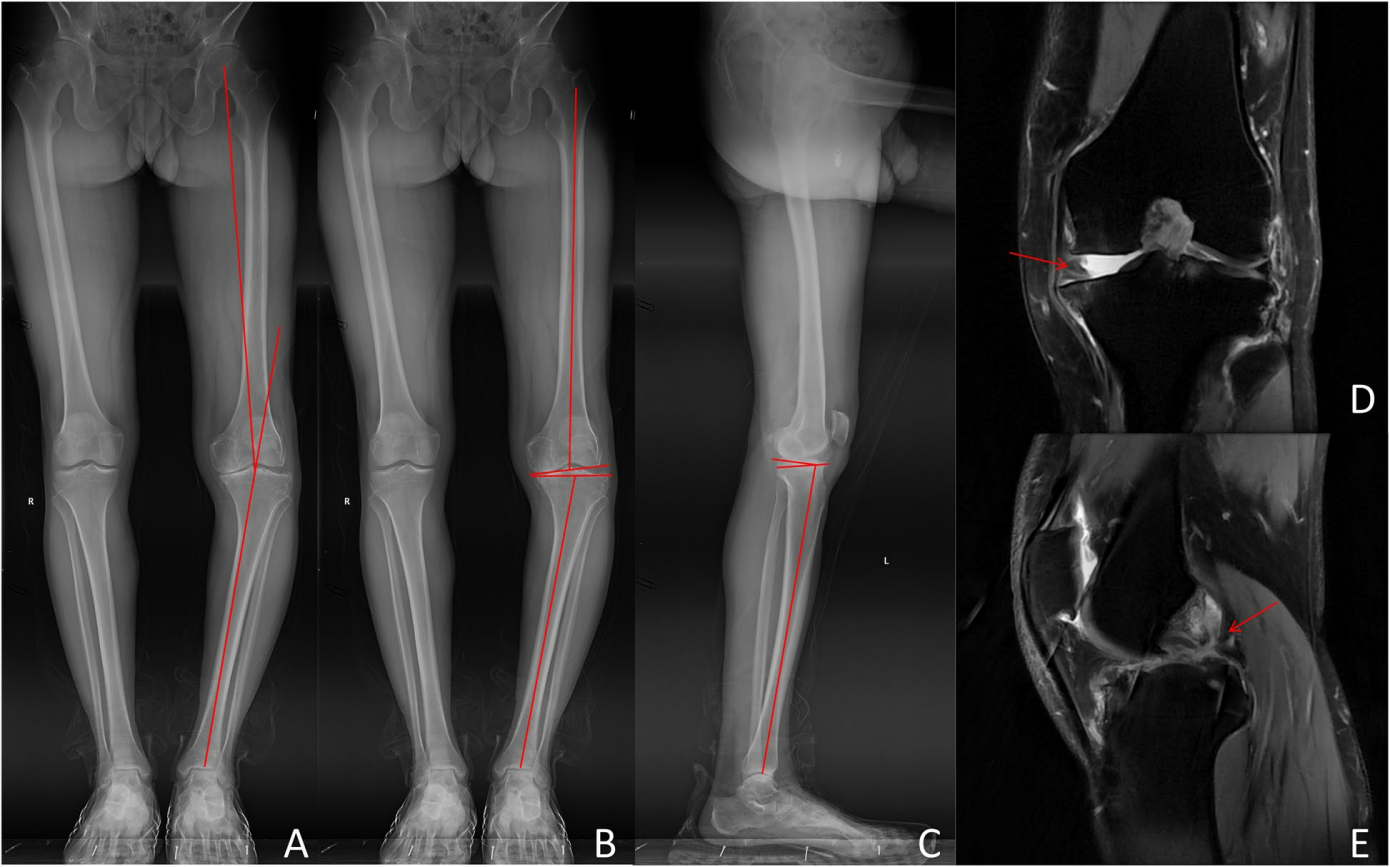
Preoperative imaging data of the patient. The hip-knee-ankle angle was 13.6° (A), the lateral distal femoral angle was 83.4° (B), the medial proximal tibial angle was 80.5° (B), the posterior inclination of the tibial plateau was 11.5°, and the joint line convergence angle was 6.0° (C). MRI revealed complete stripping of the medial cartilage (D) and rupture of the posterior cruciate ligament (E).
